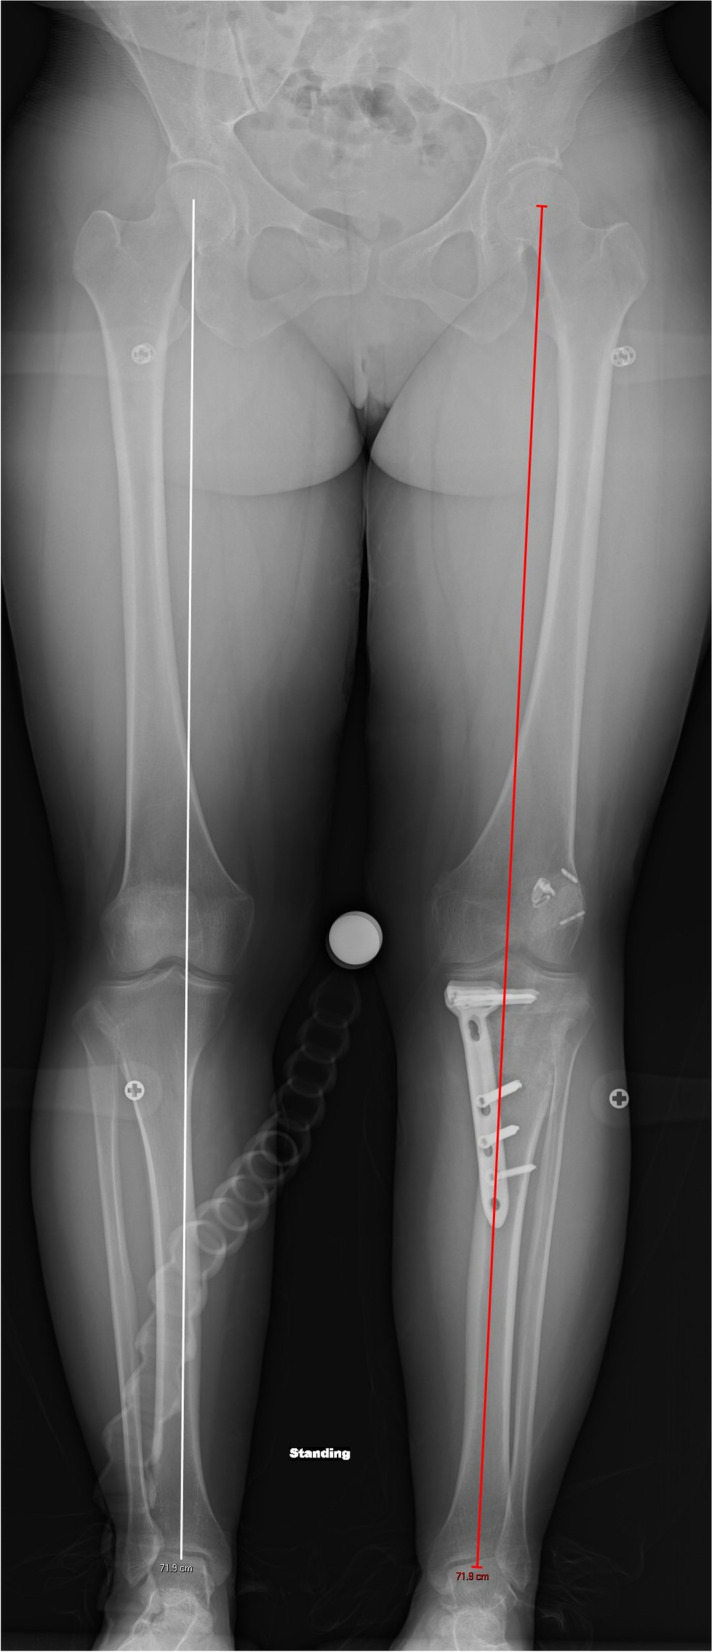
A post-operative standing mechanical axis radiograph after revision osteotomy and ACL reconstruction demonstrating neutral alignment
